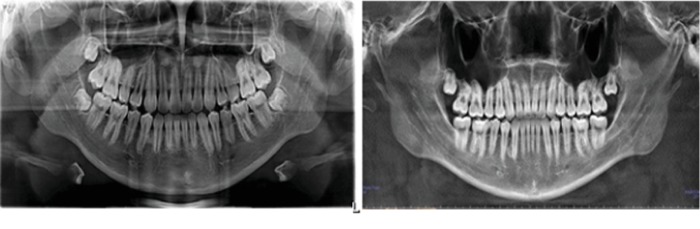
CBCT before and after treatment.
